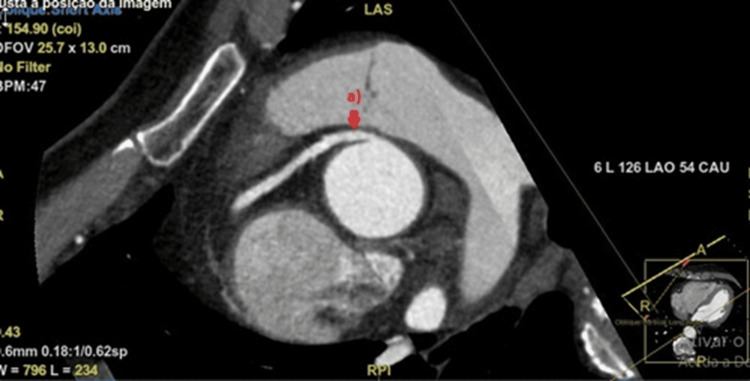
Axial slice of CCTA showing the right coronary artery (arrow) with an aortic origin at the level of the aortic wall between the right and left coronary sinuses, traveling between the aorta and pulmonary artery (interarterial course). CCTA, coronary computed tomography angiography
